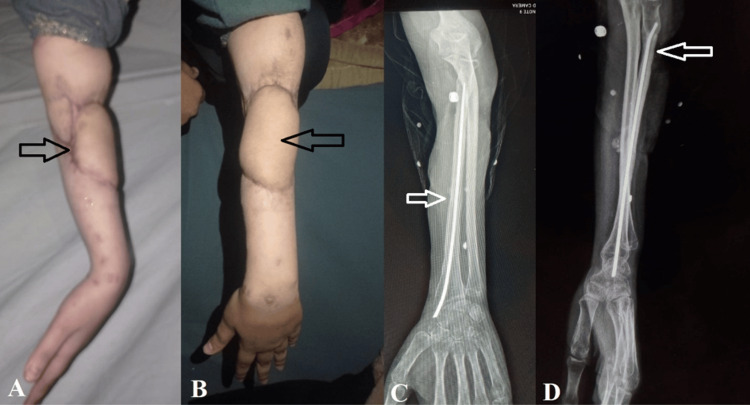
Final clinical outcome and radiographic bone healing (A, B) Four-month follow-up clinical photographs demonstrating complete soft-tissue healing and the final appearance of the affected forearm. (C, D) Six-month follow-up radiographs in anteroposterior (C) and lateral (D) views demonstrating complete graft incorporation with satisfactory bone healing and maintained alignment.
